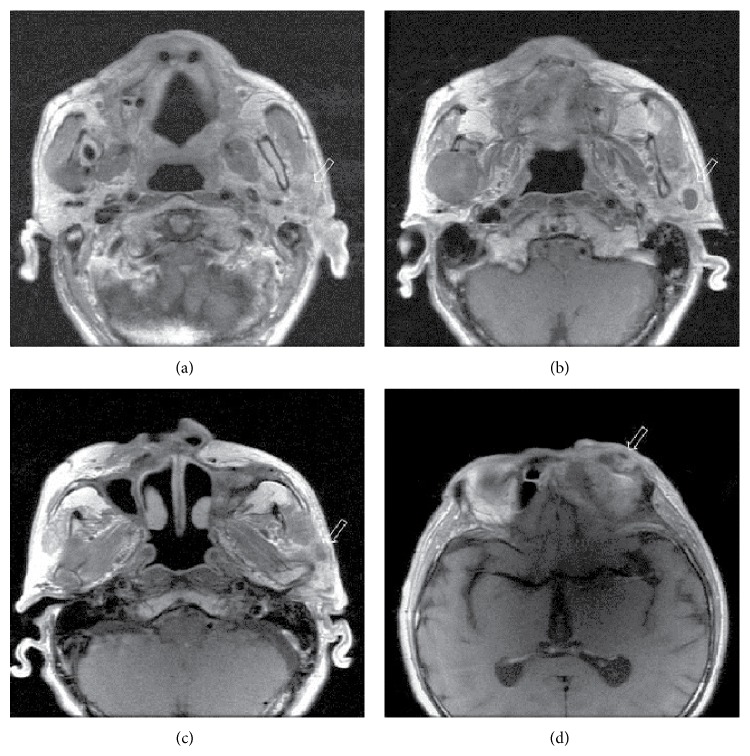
Contrast enhanced axial T1-weighed MR imaging: (a), (b), and (c) a heterogeneous soft tissue mass with cystic-necrotic components at left parotid region with extending auricula and external ear tract (light arrow mark). (d) A heterogeneous soft tissue mass with cystic-necrotic components at orbital roof with destruction of frontal bone (light arrow mark).
